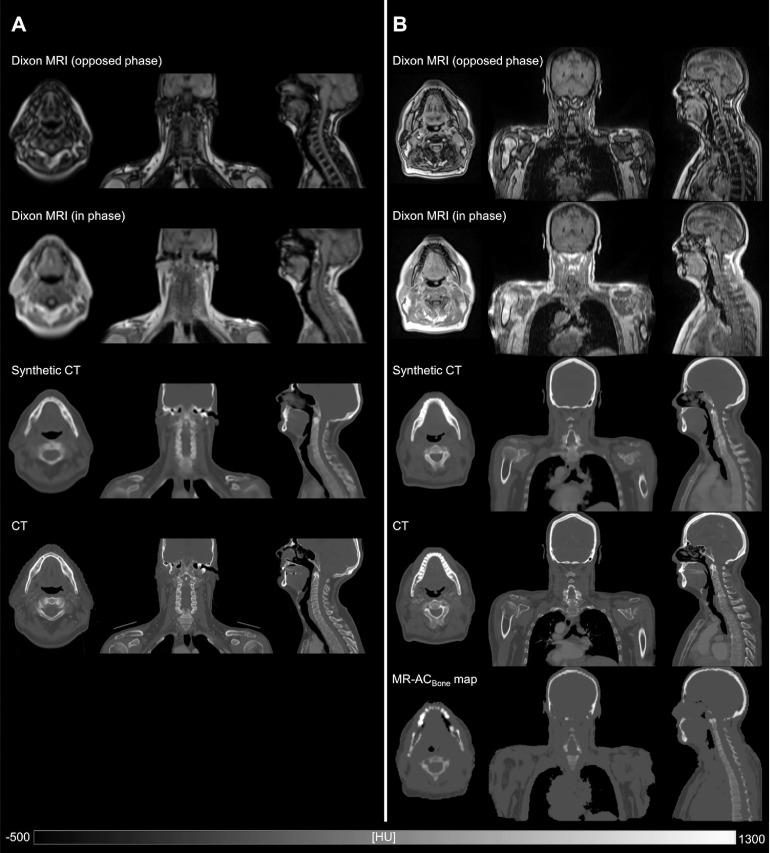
A patient example from the external cohort (A) and the local cohort (B). From top to bottom: The water and fat opposed-phase and in-phase Dixon magnetic resonance imaging (MRI), which serve as model input. The synthetic computed tomography derived from either the original model (A) or the updated model (B). The reference computed tomography. The MR-AC_Bone_ map (only in B). Notice the improved MRI resolution and the increased axial field-of-view for B compared with A.
